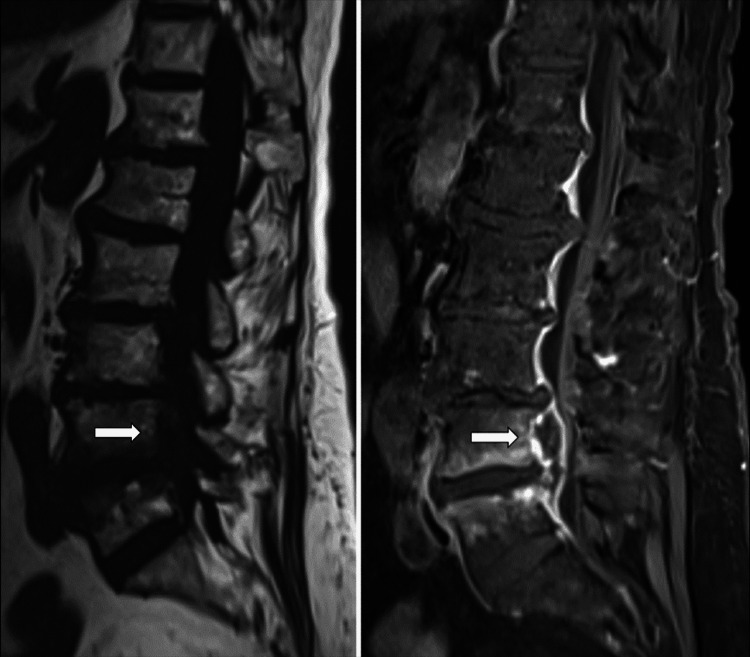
MRI with and without contrast evidenced the epidural abscess and spondylitis
